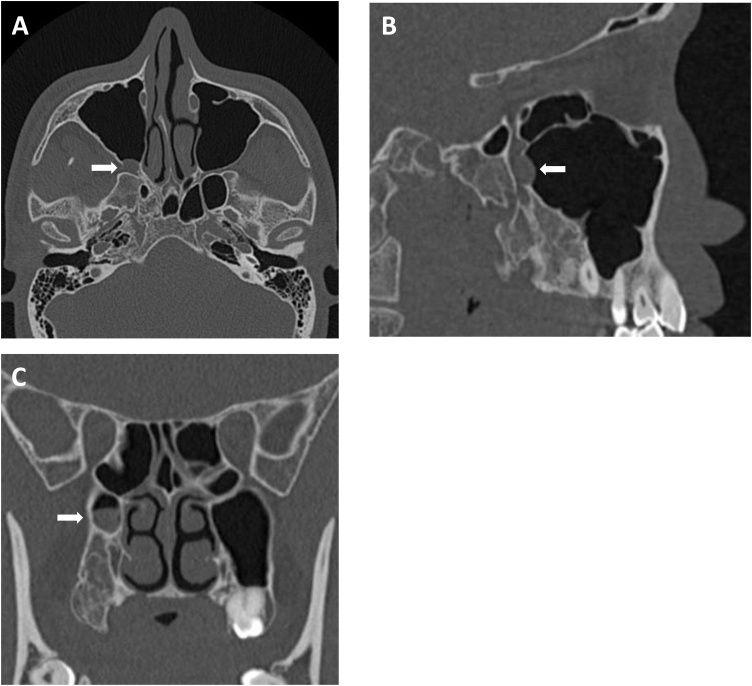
CT scans show recurrent OKC in the posterior wall of the right maxillary sinus (arrows). (A) Axial scan. (B) Sagittal scan. (C) Coronal scan.
